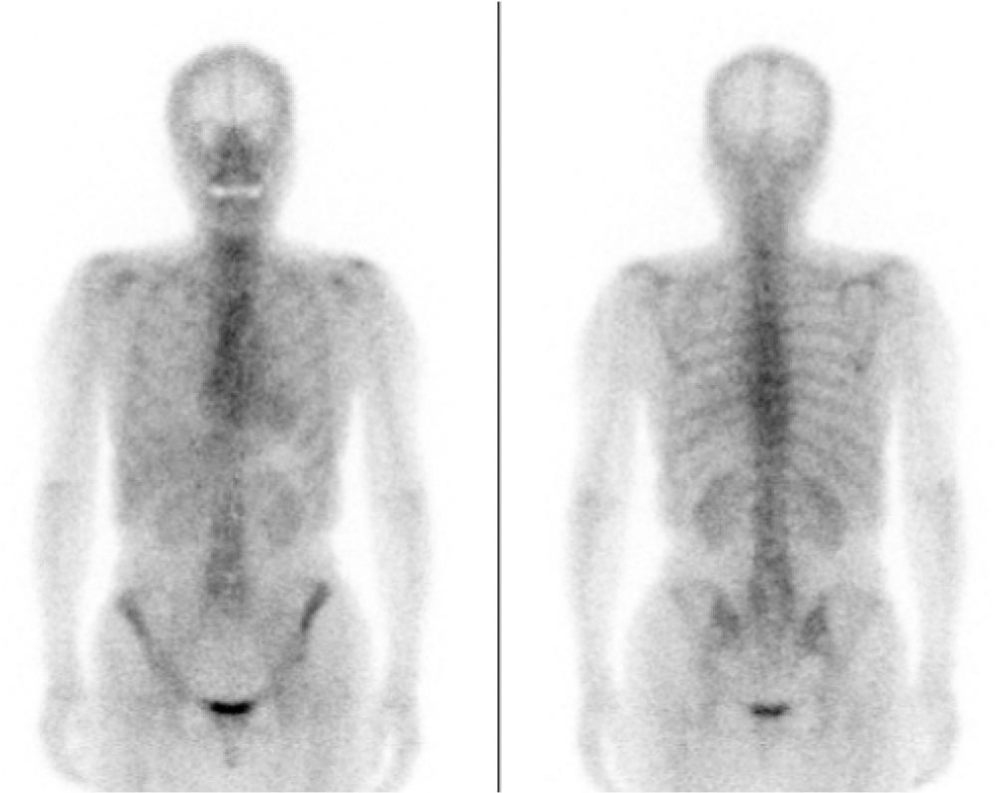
Technetium-99m pyrophosphate scintigraphy demonstrated mild myocardial uptake (heart-to-contralateral ratio = 1.26, Grade 1), less intense than rib uptake, consistent with a negative finding for cardiac amyloidosis. H/CL, heart-to-contralateral.
